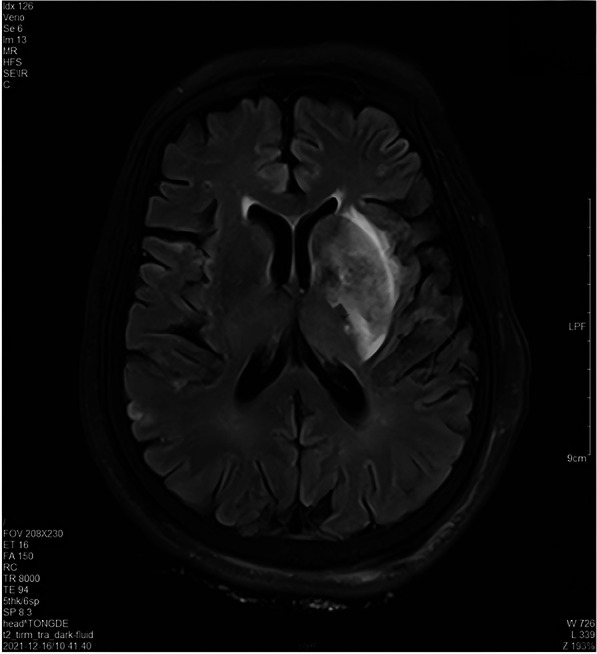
Cranial MRI on December 16, 2021.
